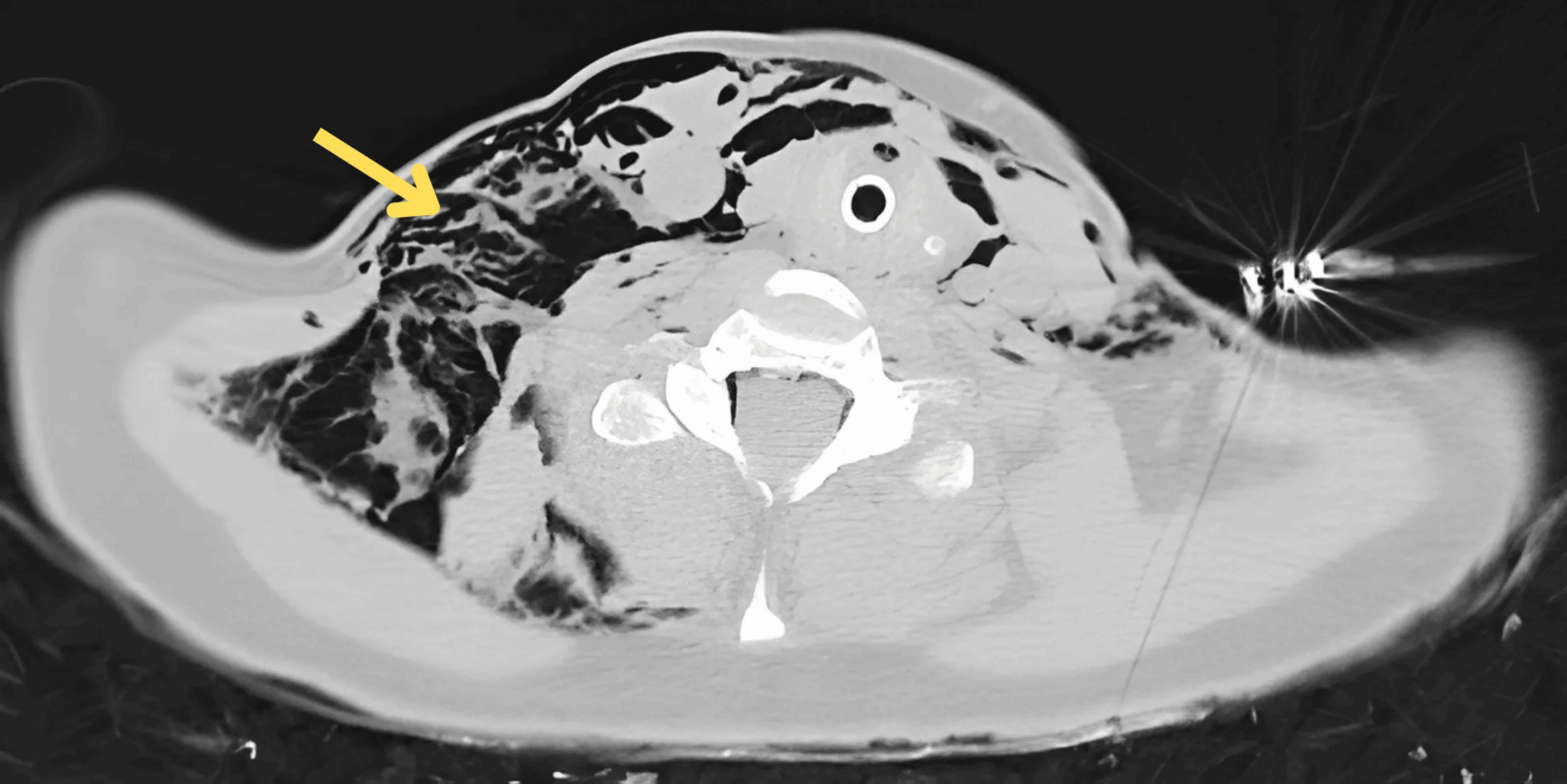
CT image (axial): cervical subcutaneous emphysema (arrow).
